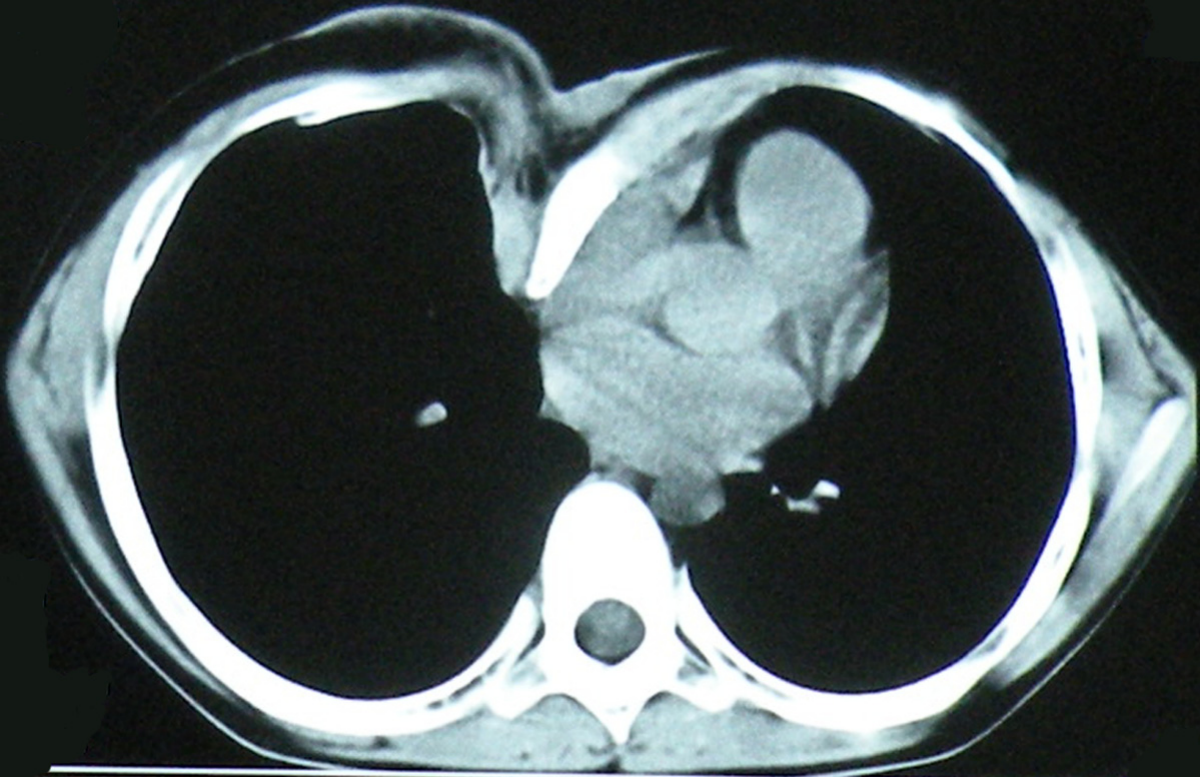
At the level of sixth thoracic vertebra, CT scan shows marked sternal rotation (the sternum is rotated by almost 90 degrees) and depression, with a pectus index of 3.9.
